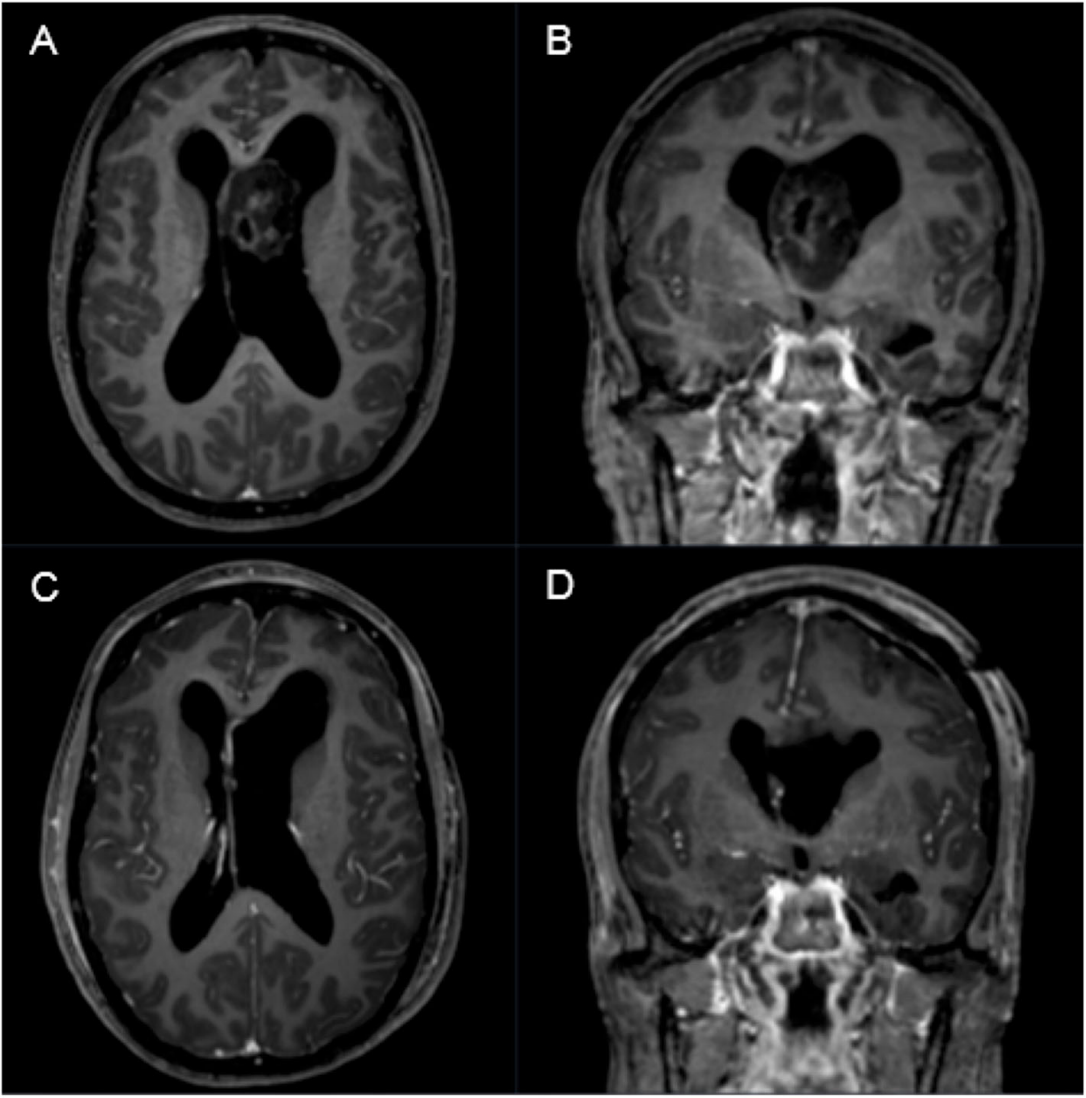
A 44-year-old female patient presented with aggravating headache, vertigo, gait disturbance and concentration disorder in sense of hydrocephalic symptoms for 3–4 months. a, b Preoperative T1-weighted gadolinium enhanced MRI showing a low to intermediate heterogeneously enhancing intraventricular mass in the third ventricle with consecutive secondary hydrocephalus. c, d Postoperative T1-weighted gadolinium enhanced MRI showing complete removal of the tumor through a left frontal precoronary transventricular keyhole approach. Pathological findings confirmed WHO grade I subependymoma
